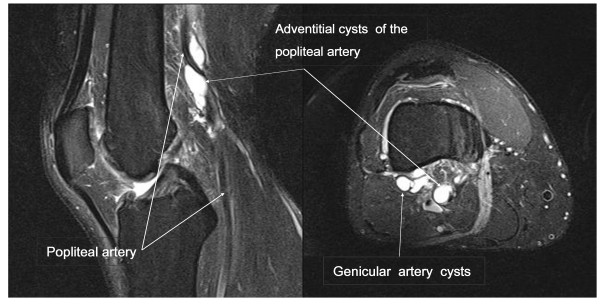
T2-weighted image of our patient's magnetic resonance imaging scan. The sagittal image on the left demonstrates one cyst in the posterior wall of the popliteal artery and a further anterior wall cyst causing stenosis. The axial image on the right shows the popliteal and genicular artery cysts. Lucent areas represent the arterial lumen.
